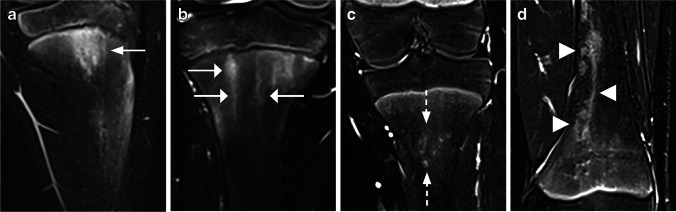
Coronal water-only Dixon T2-weighted magnetic resonance images in (a) an 11-year-old boy with a roundish area of increased signal intensity in the metaphysis of the tibia (*arrow*), (b) an 11-year-old boy with multiple areas of linear-shaped increased signal intensity in the metaphysis of the tibia (*arrows*), (c) a 12-year-old girl with an area of punctuated high signal intensity in the metaphysis of the tibia (*dotted arrows*) and (d) a 13-year-old girl with an area of increased signal intensity in the femur metaphysis with a mixed roundish and linear shape *(arrowheads*)
